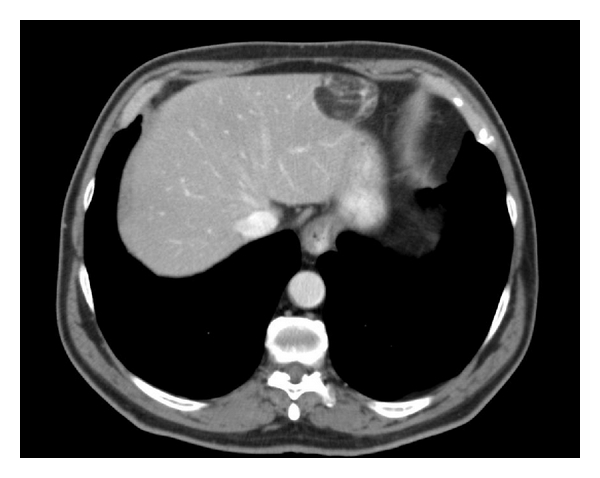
Abdominal CT showing a peripheral lipomatous lesion in left lateral sector.
